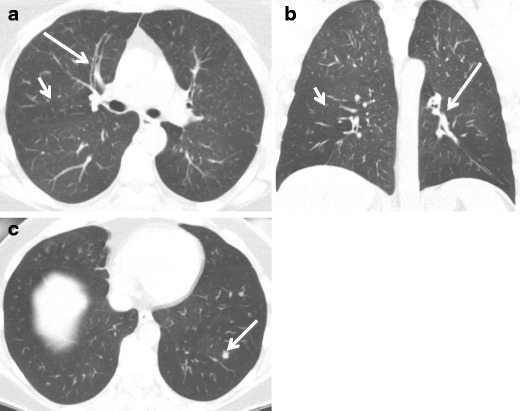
Fifty-one-year-old woman with a axial and b coronal CT images demonstrating bronchial wall thickening and obstruction (*long arrows*) and areas of mosaic attenuation consistent with regions of air trapping (*short arrows*). There are also c groundglass nodules (*arrow*) consistent with bronchiolitis
